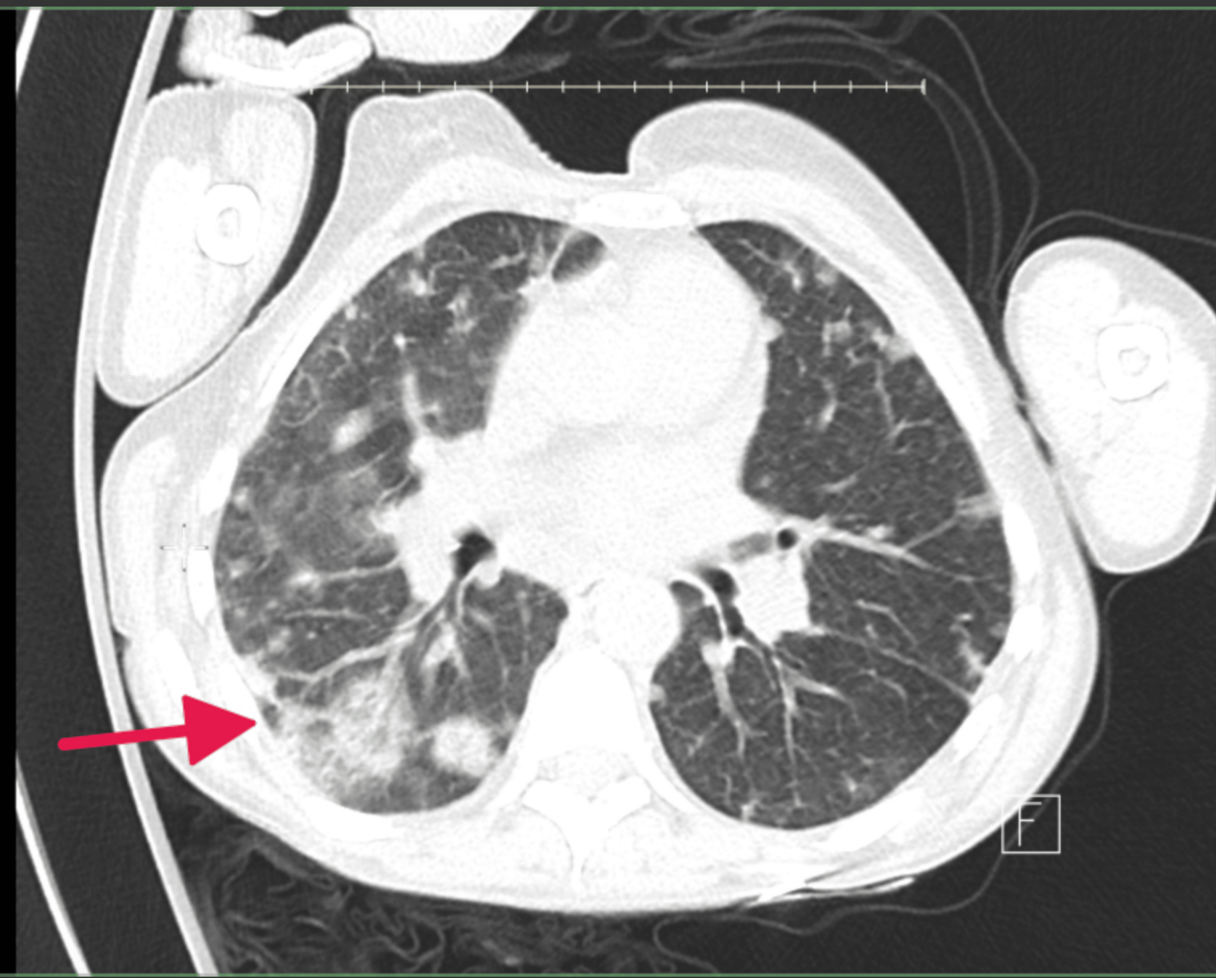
CT angiography of the chest and abdomen: bilateral septic emboli at the time of admission.
